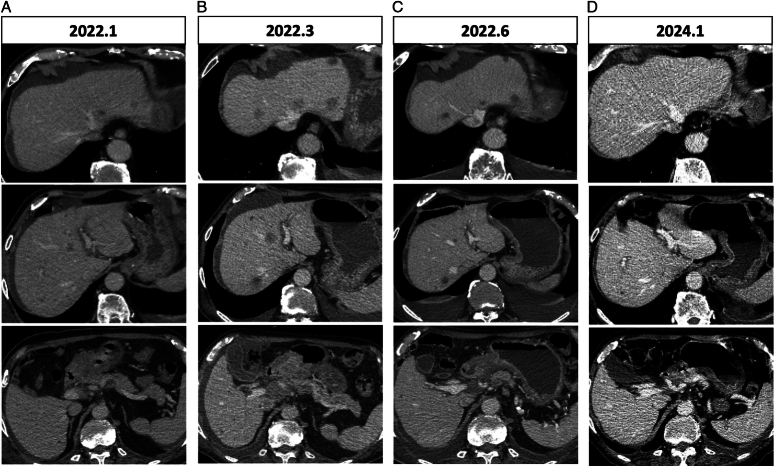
CT imaging during the clinical course. (A) Baseline image before the initiation of trastuzumab treatment. Hepatic nodules and antrum mass can be observed. (B) After one cycle of trastuzumab treatment, tumor progression was evidenced by increased tumor size. (C) After two cycles of the combination treatment of pembrolizumab and trastuzumab, the tumor began to shrink. (D) Latest CT image taken after 21 months of combined treatment with trastuzumab and pembrolizumab showed significant tumor reduction.
